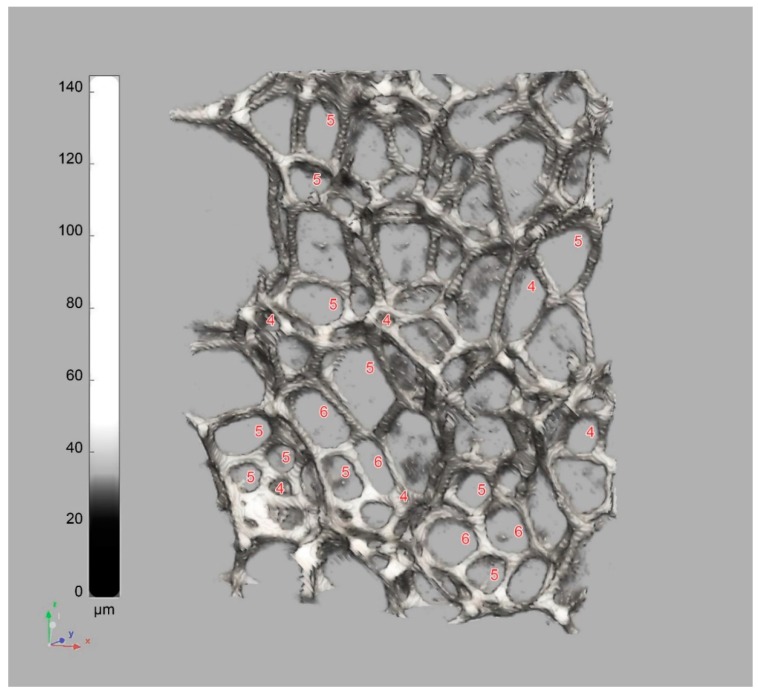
The PU foam microstructure, a cutout from the reconstructed W-foam sample (µ-CT resolution 6.25 µm). The cell faces are labeled with numbers of their edges. The greyscale corresponds to strut thickness.
